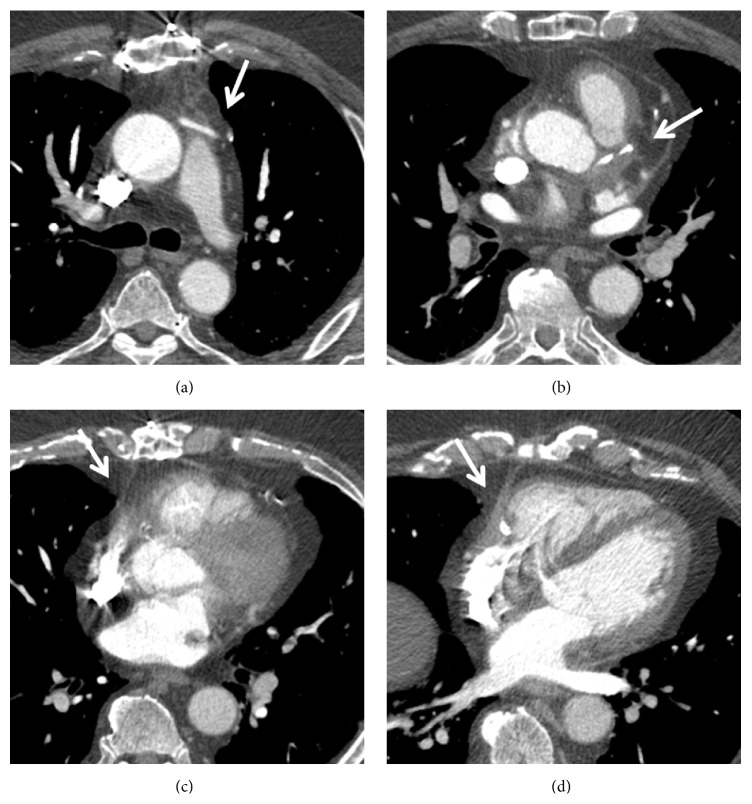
Contrast-enhanced computed tomography with subsequent images through the heart, in patient with granulomatosis with polyangiitis, shows resolution of soft tissue attenuation around the coronary artery graft (a), left main coronary artery (b), and right coronary artery (c, d) after the immunosuppressive therapy.
